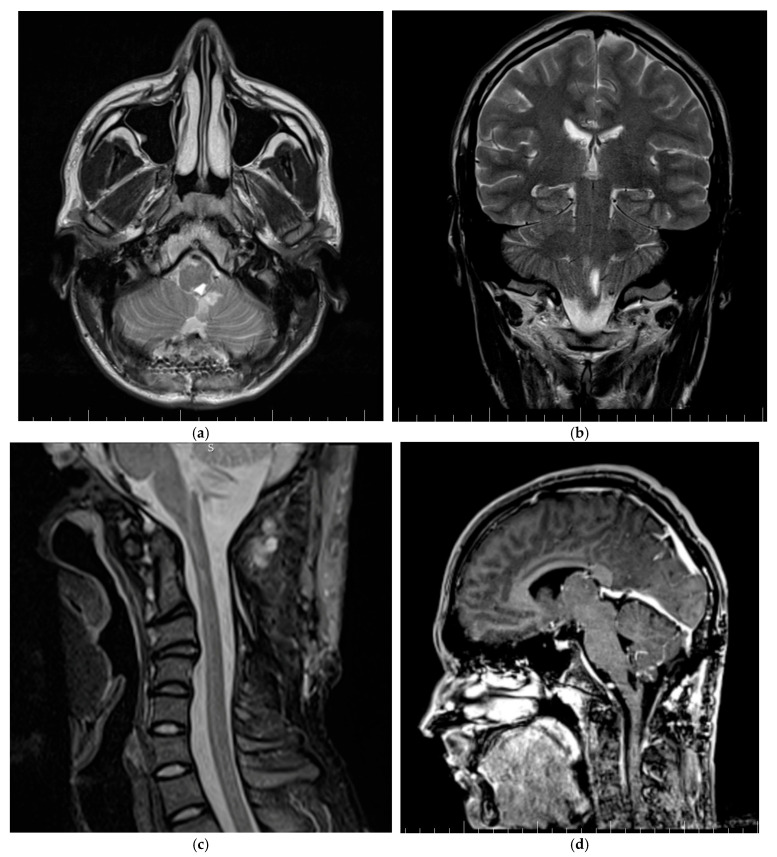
Postoperative MRI examination of patient three after complete excision of a brain stem hemangioblastoma. (a) Axial section, T2-weighted sequence, highlighting the complete remission of the cyst. (b) Coronal section, T2 sequence highlights the complete evacuation of the cyst and the presence of a post-resection cavity of the mural nodule. (c) sagittal section, TIRM sequence. (d) Sagittal section, T1 postcontrast sequence that highlights the complete resection of the mural node.
